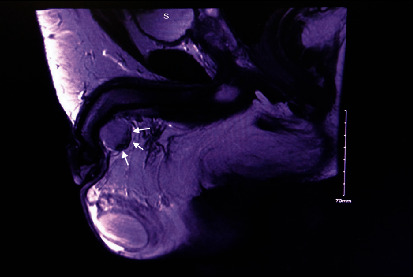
Magnetic resonance imaging finding of a mass in the left inferolateral side of the base of the penis, measuring 3.1 cm in maximum dimension.
